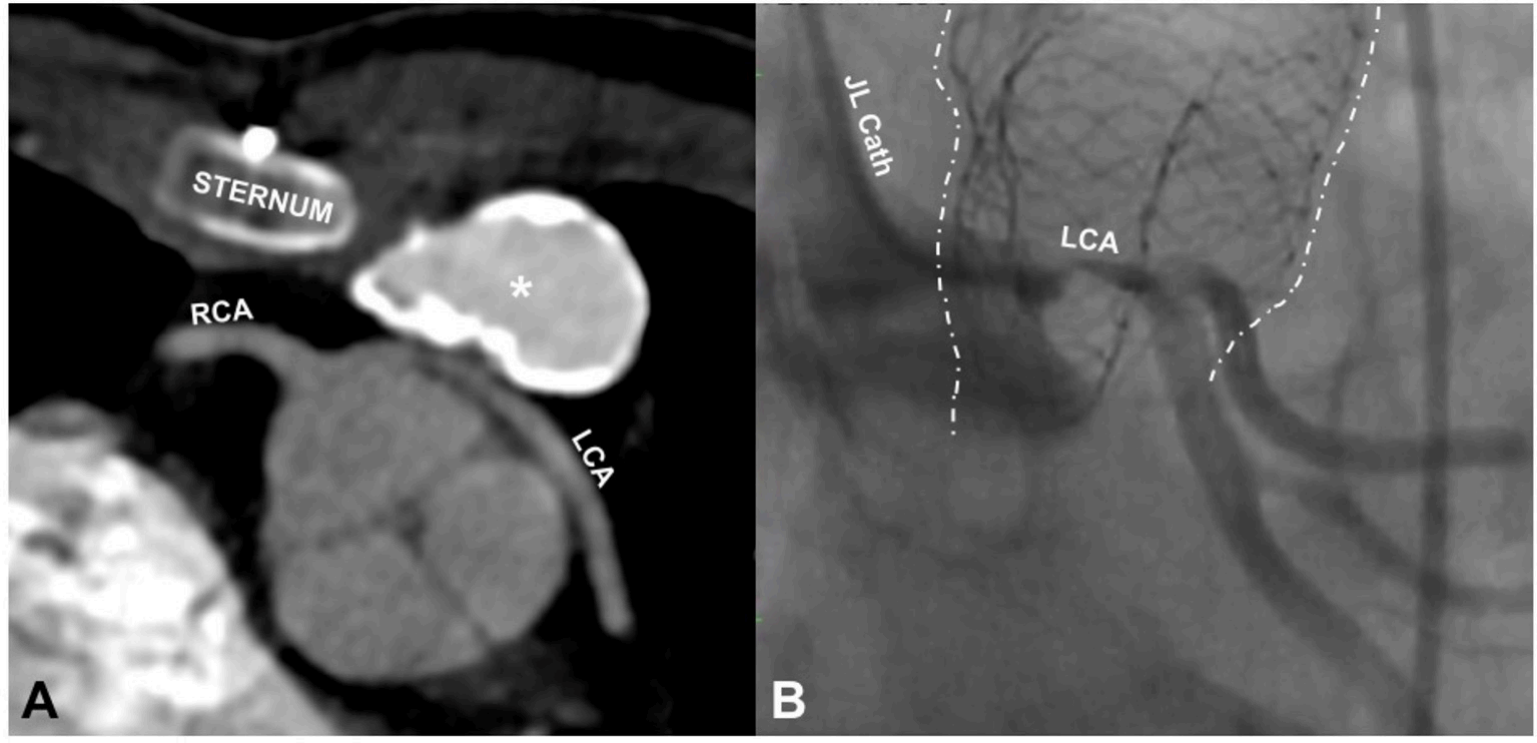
Coronary compression. (A) is an axial slice from the CT scan of a 14 year old male showing compression of the left main stem coronary artery (LCA) by a 20 mm Contegra conduit (*), placed 6 years before the CT scan. The origin of this coronary is abnormal, arising from the right coronary sinus beside the right coronary (RCA) before wrapping around the aortic route. The coronary anomaly and the important distortion of the coronary were unrecognized until the patient presented with ventricular tachycardia during a basketball game. (B) shows a left coronary angiogram in a different patient (30 year old male) taken during cannulation for ECMO following compression of the left coronary by an outflow tract stent (dotted white lines) placed in preparation for a pulmonary valve implant. The stent is distorted, having been partially crushed by chest compressions after haemodynamic collapse. The coronary label (LCA) is placed directly above the area of near total occlusion. The patient had a surgical valve replacement and was discharged home 5 days later.
